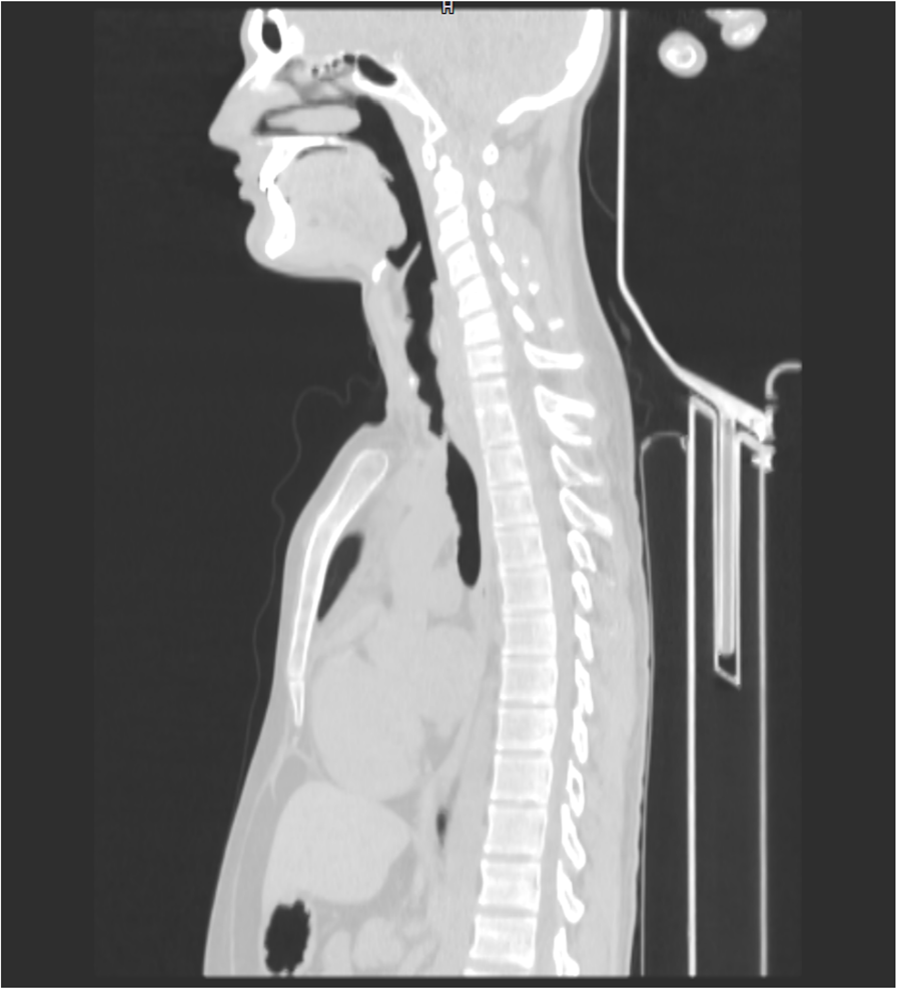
CT scan of tracheal stenosis
